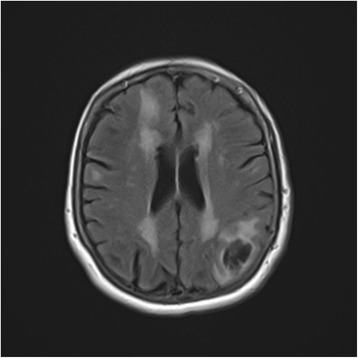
Magnetic resonance imaging of the brain was remarkable for multiple hemorrhagic ring-enhancing lesions with surrounding vasogenic edema, highly suspicious for hemorrhagic metastases versus abscesses
